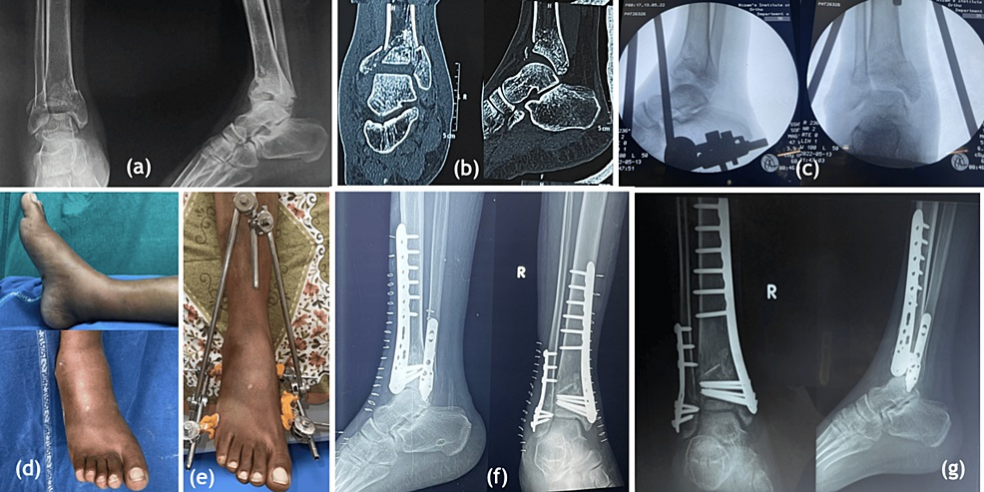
Staged management with initial spanning external fixator application followed by definitive fixation. (a) AP and lateral radiographs on presentation, (b) CT scan showing impaction of the articular surface on coronal and saggital sections, (c) AP and lateral radiographs post spanning external fixator application, (d) swelling on day of presentation, (e) appearance of wrinkles on day 3 of application of external fixator, (f) AP and lateral radiographs immediately after definitive fixation with medial distal tibial locking plate and distal fibula anatomical plate with tricortical bone grafting, (g) AP and lateral radiographs after 3 months of definitive fixation. AP: anteroposterior; CT: computer tomography
